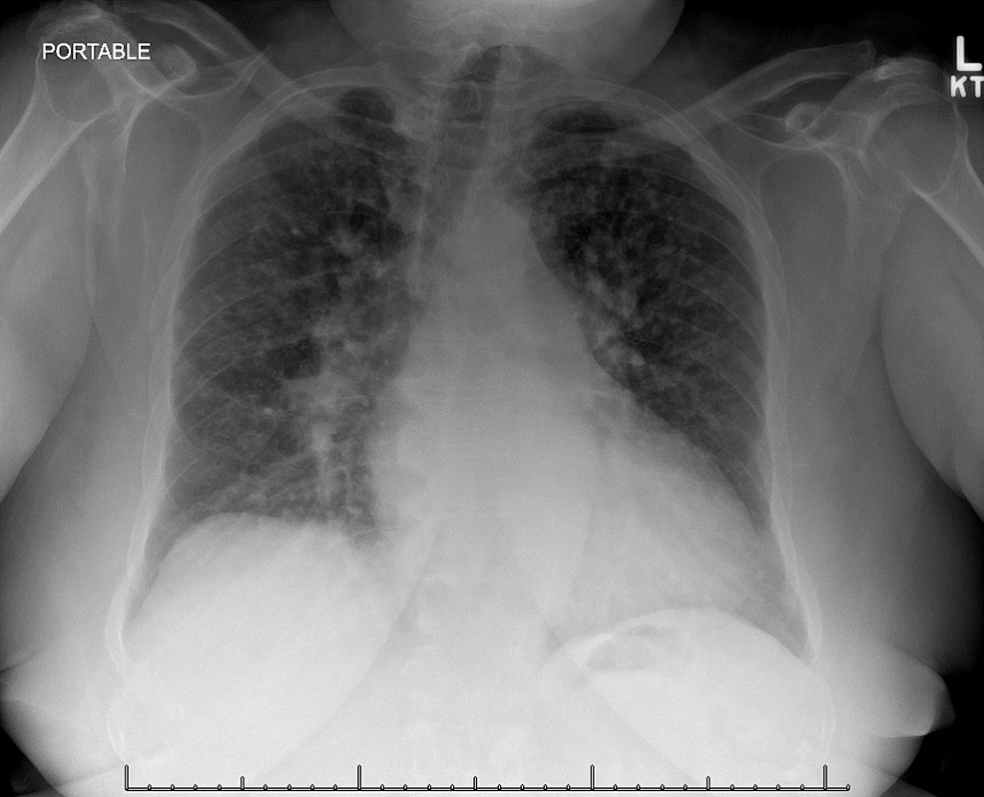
Chest radiograph showing reticulonodular opacities in both lung fields
